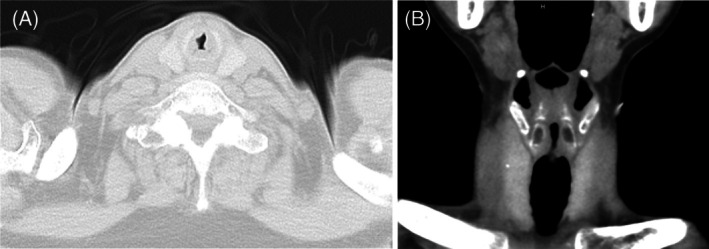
Computed tomography reveals airway stenosis due to thickening of the subglottic lesion: (A) axial view, (B) coronal view. No pulmonary parenchymal involvement is observed.
